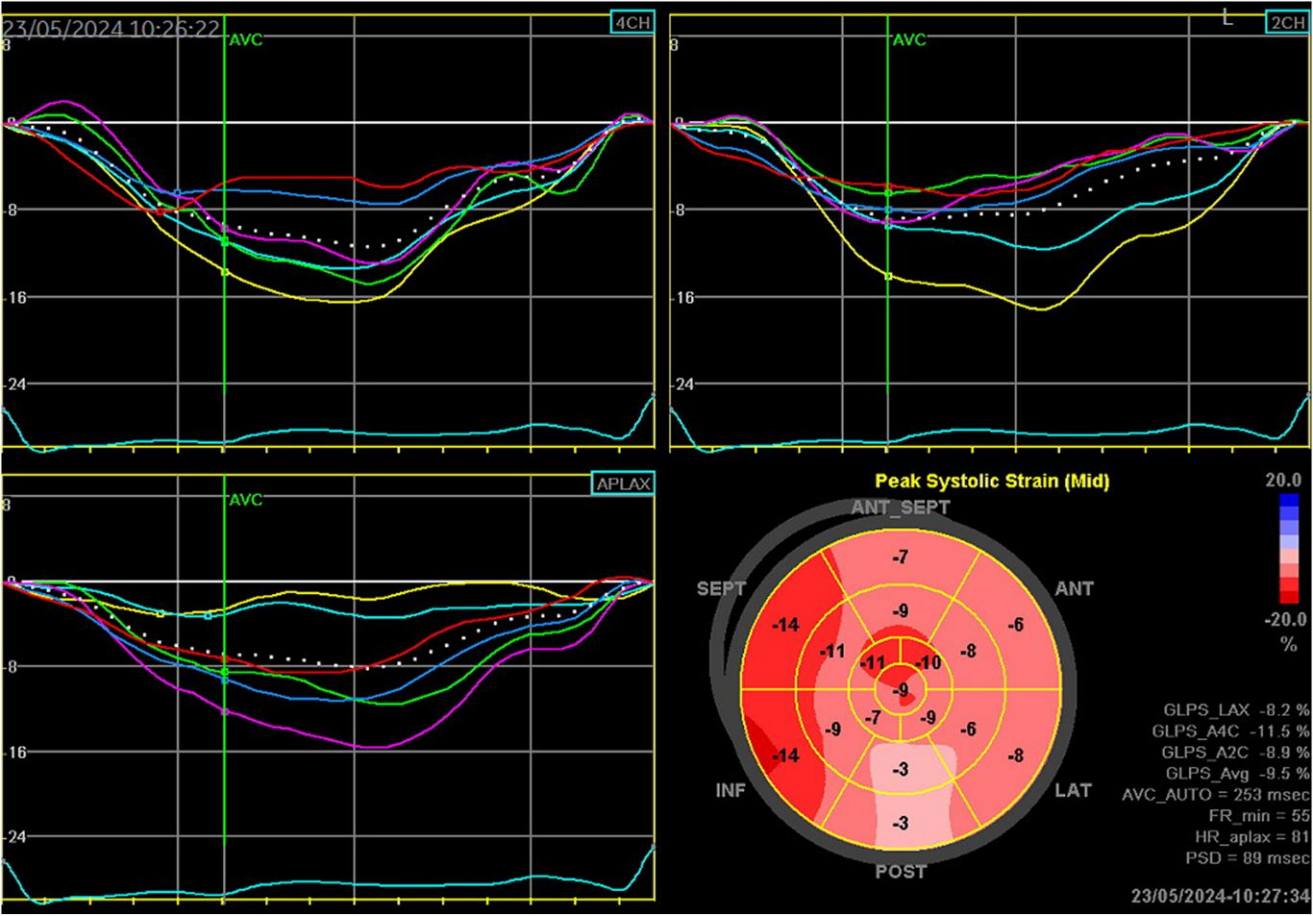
The patient’s GLS, assessed by speckle-tracking echocardiography, was calculated as −9.5%. No apical sparing pattern was observed.
